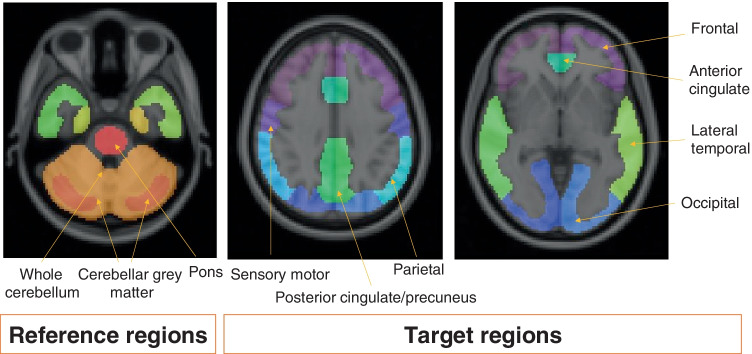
Example of the most common reference and target regions used when generating SUVr
